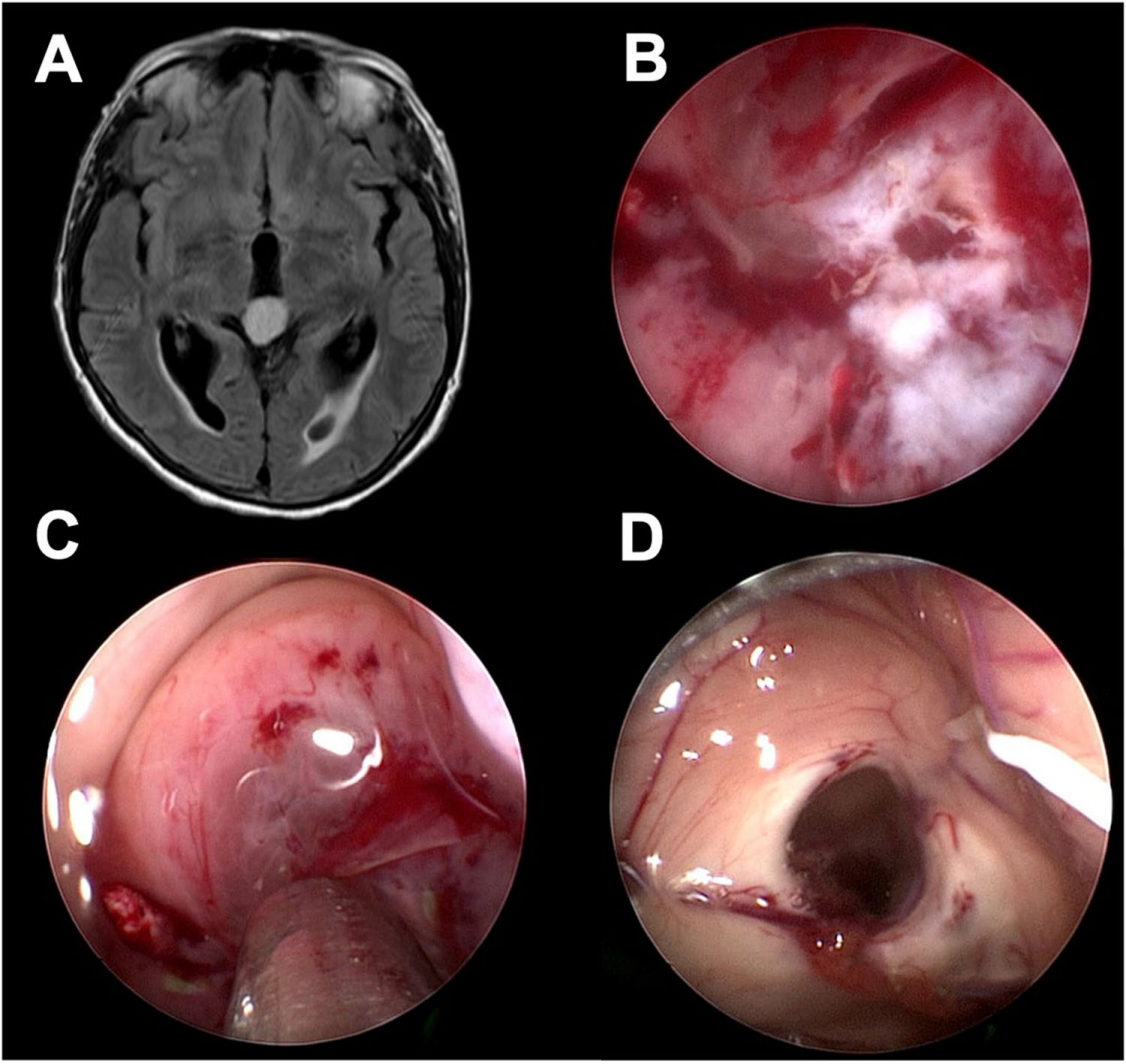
Example of the application of the dry field technique. Preoperative MRI of a 74-year-old patient with anaplastic astrocytoma at the pineal region (A). The patient was scheduled for an ETV, tumor biopsy and optional partial resection. The tumor was well vascularized and the surgeon decided to proceed with the procedure under dry field condition (B). A partial resection was performed using a endoscopic ultrasound aspirator (C). The inspection of the ventricle at the end of the procedure showed no ventricle collapse (D). The ventricle system was refilled and no postoperative complications occurred
